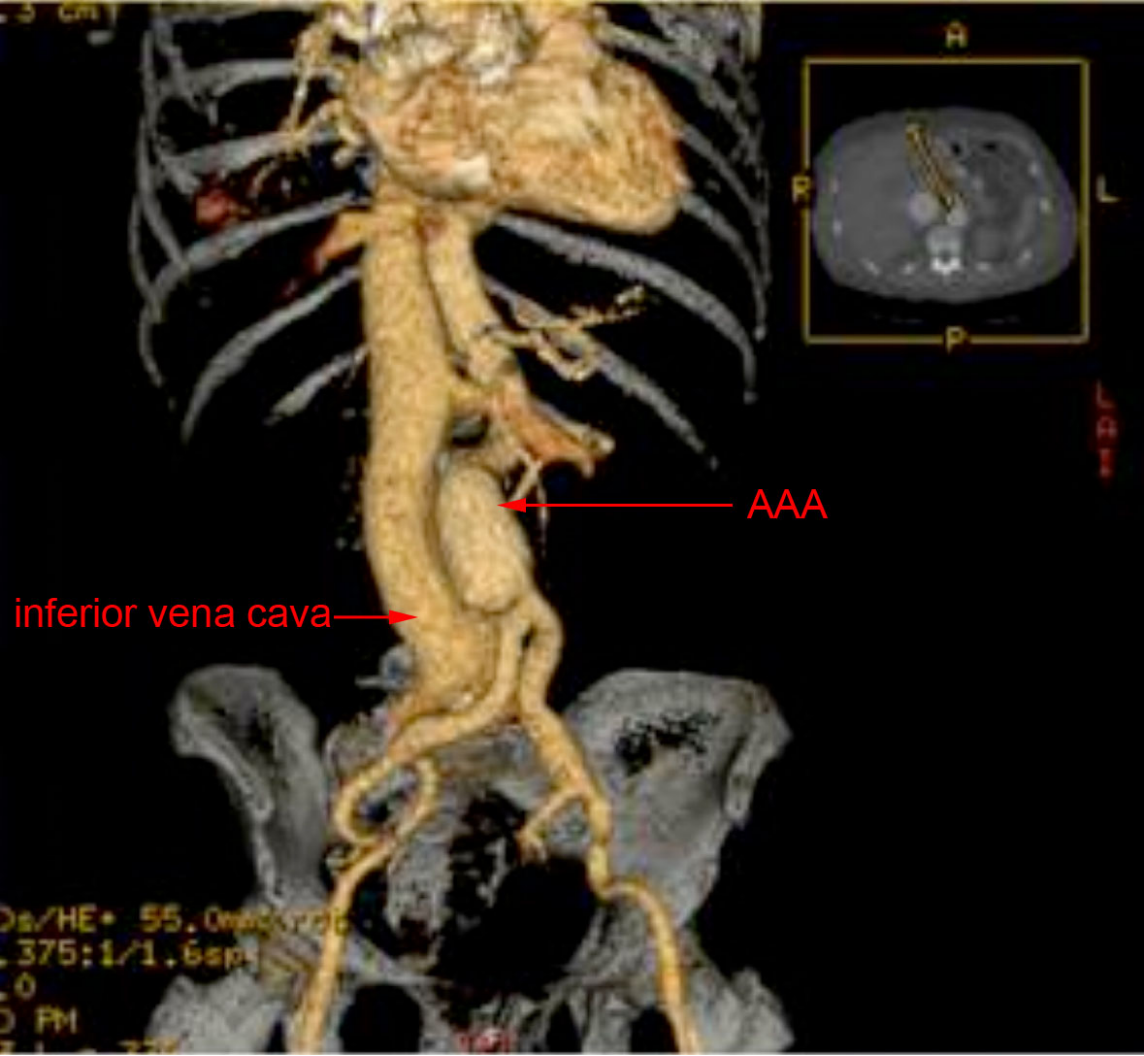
3D contrast CT showing abdominal aortic aneurysm and the dilated inferior cava vein.
